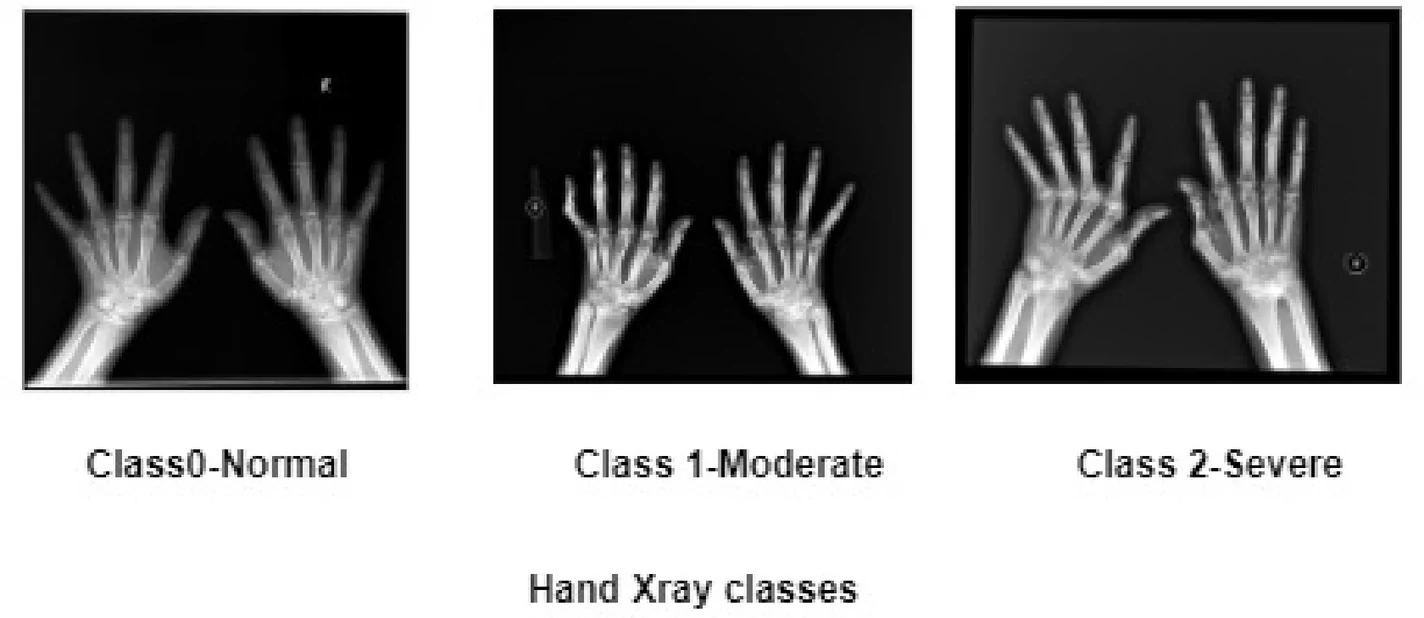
Different types of classes: Hand X-ray.
